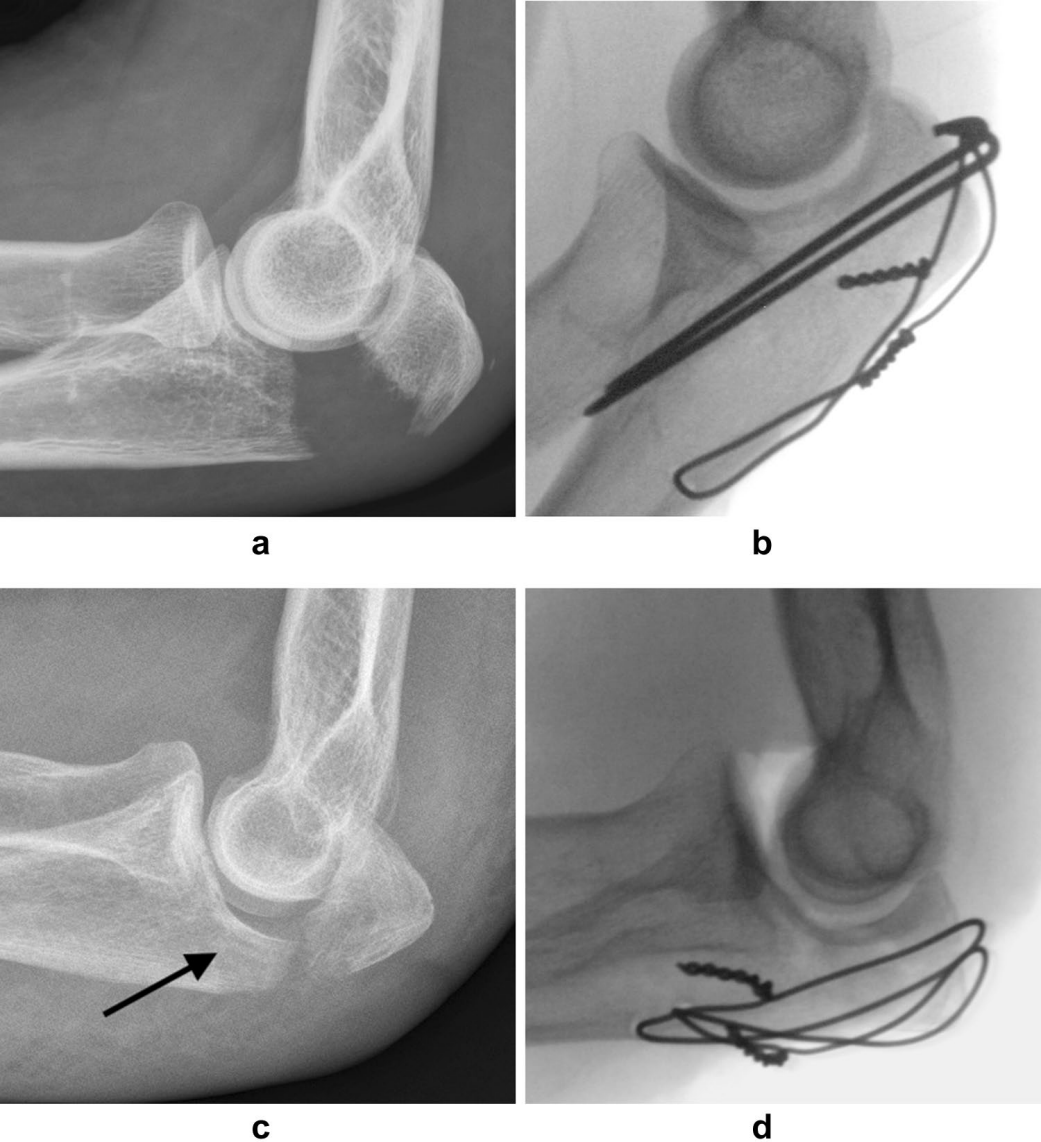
a Pre-operative lateral radiograph of a Mayo class 2a olecranon fracture. b Tension band wiring. Intraoperative lateral radiograph of the fracture in a after reduction and tension band wire fixation. The cerclage is proximally anchored in the bend of the K-wires. c Pre-operative lateral radiograph of a Mayo class 2b olecranon fracture. There is a depressed central fragment (arrow). d Cerclage fixation. Intraoperative lateral radiograph of the fracture in c after open reduction and fixation with two cerclages. The cerclages are in figure-8 and figure-0 configuration, respectively, and are proximally anchored in the triceps tendon
